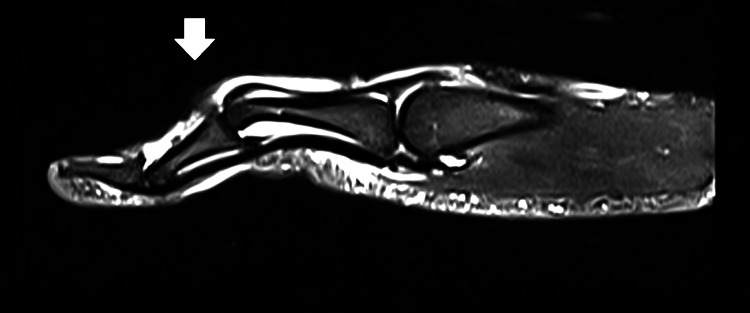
Magnetic resonance image of the fifth metacarpal joint Imaging revealed a tear of the central slip of the extensor tendon (arrow), along with a boutonniere deformity and volar subluxation of the middle phalanx at the proximal interphalangeal joint.
